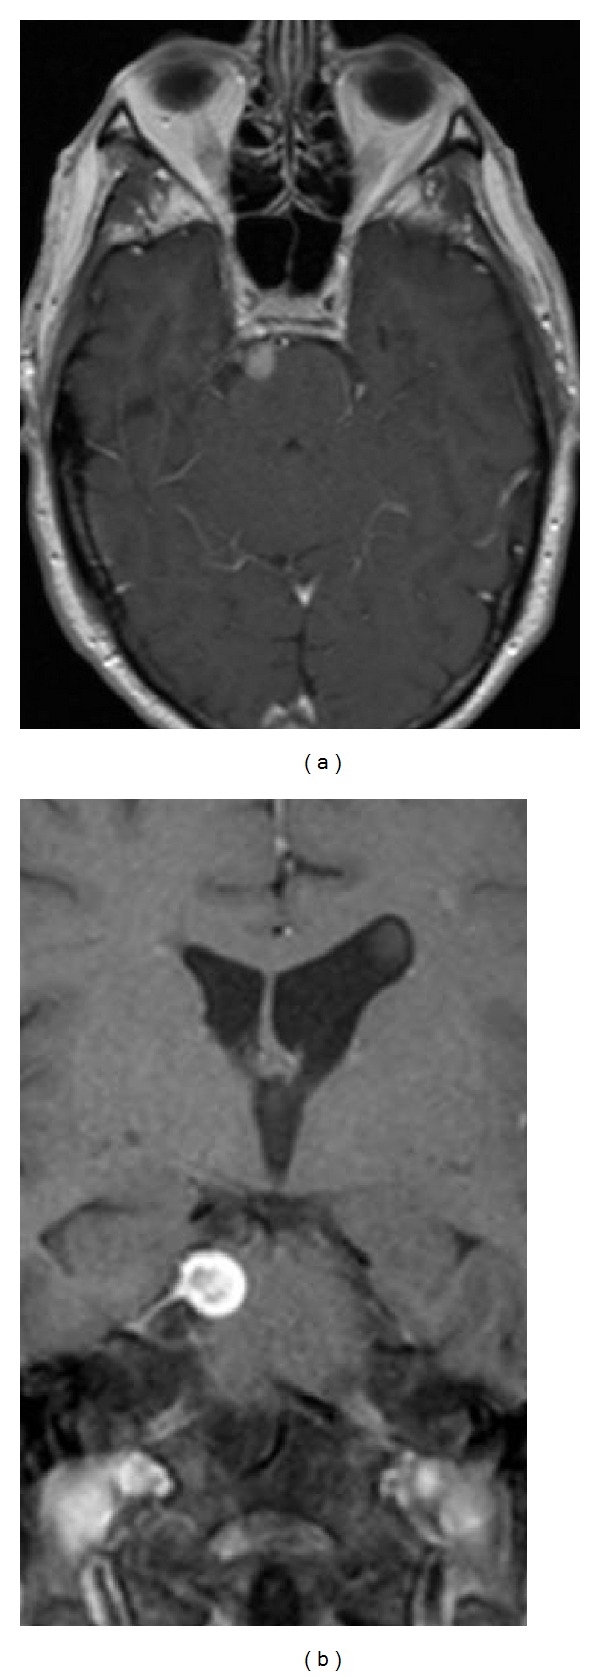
Preoperative MRI brain with contrast axial (a) and coronal T1-weighted (b) images reveals a well-circumscribed, heterogeneously enhancing lesion adjacent to the right side of the upper pons.
